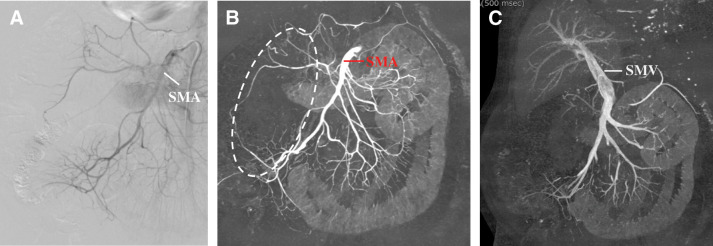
(A) Angiography findings. The SMA showed spasmic changes, and the middle colic artery, right colic artery, and ileocolic artery were poorly enhanced. (B, C) 3D-reconstructed images from the contrast-enhanced CT, which were obtained after the angiography. (B) Arterial phase. The intestinal parenchyma supplied by the middle colic artery, right colic artery, and ileocolic artery was poorly enhanced (area surrounded by the dotted white line). (C) Venous phase. The right colic vein was not enhanced. SMA, superior mesenteric artery; SMV, superior mesenteric vein
